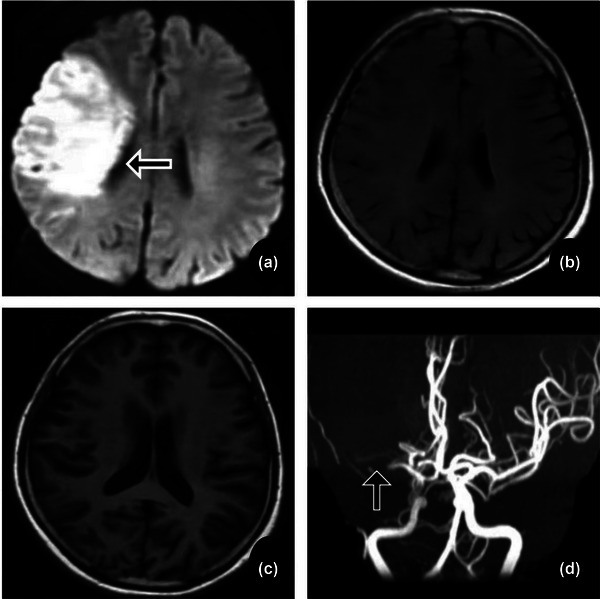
NVAF+ICAS patient. A 68‐year‐old female was admitted 2 h after stroke and she was a NVAF patient. A: DWI, B: T2WI‐FLAIR, C: T1WI, D: MRA. Massive infarct in the right cerebral hemisphere, right MCA occlusion, and right PCA atherosclerotic stenosis.
